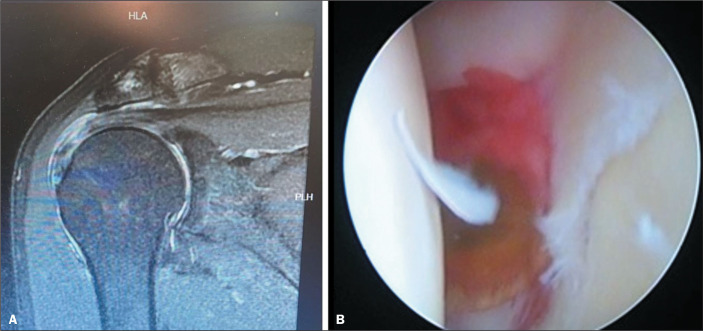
Comparison between MRI and arthroscopy for the diagnosis of a SLAP
